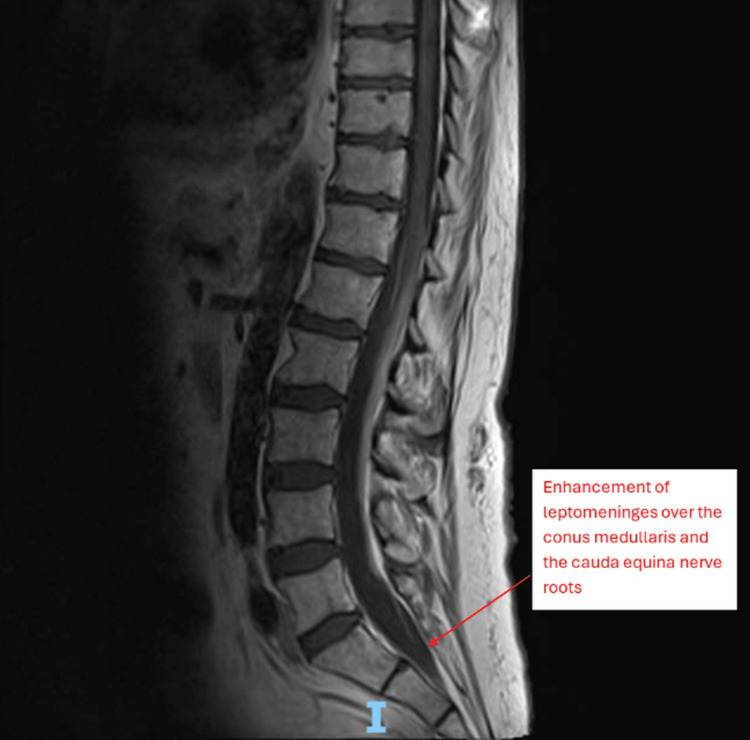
T1 sequence MRI of thoracic and lumbar spine showing leptomeningeal enhancement over the conus medullaris and the cauda equina nerve roots MRI: magnetic resonance imaging
